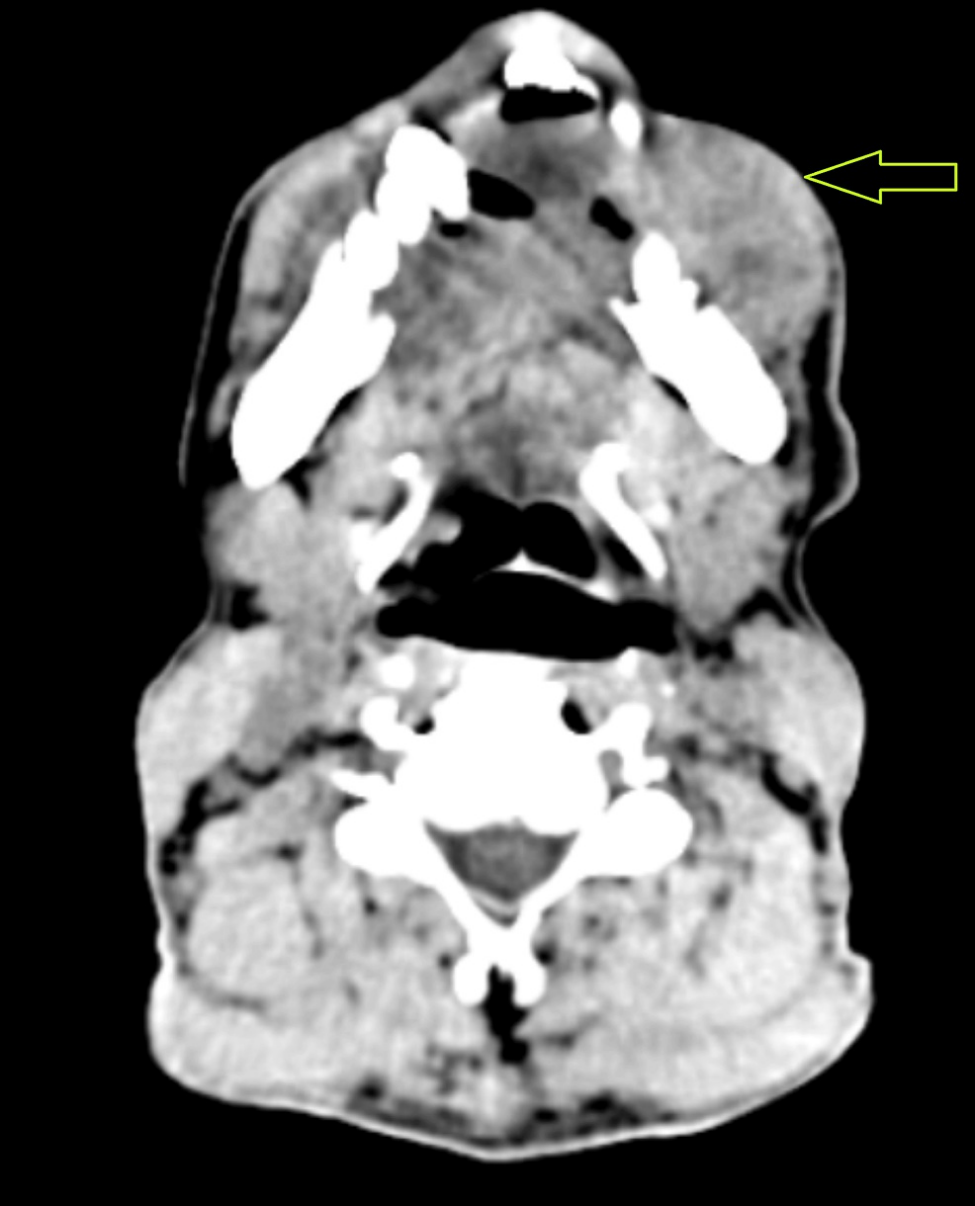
Contrast-enhanced CT showing extraoral fungating mass with no intraoral extension
